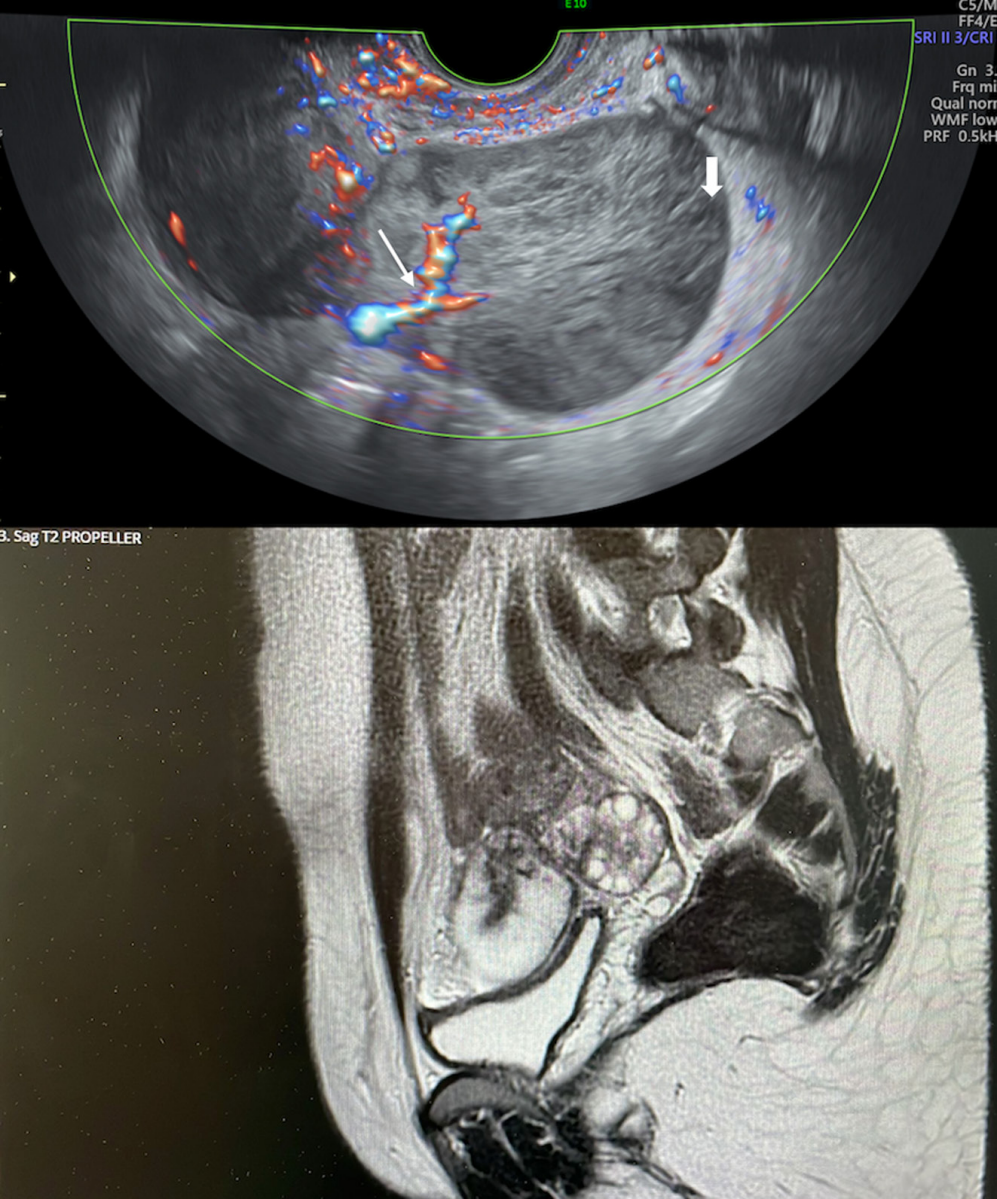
Ultrasound pattern of case 2 (heterogeneous parenchyma without acoustic shadows, with follicles in the periphery, thick arrow, and a central vascular axis color score 2, thin arrow) and corresponding magnetic resonance imaging (enlarged ovary with haemorrhagic component).
